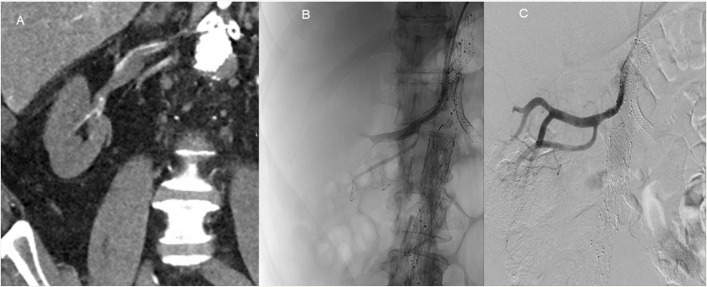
A 68-year-old patient was treated with BEVAR due to a contained rupture of a type IV aortic aneurysm. Baseline eGFR was 88 mL/min. Four months later, the patient was admitted with anuria and back pain lasting more than 24 h. Contrast-enhanced CT showed bilateral renal artery thrombosis. The left renal artery could not be recanalised. The right renal artery was treated with local thrombolysis with a good result (A-C), and the patient is not on dialysis, with an eGFR of 40 mL/min
